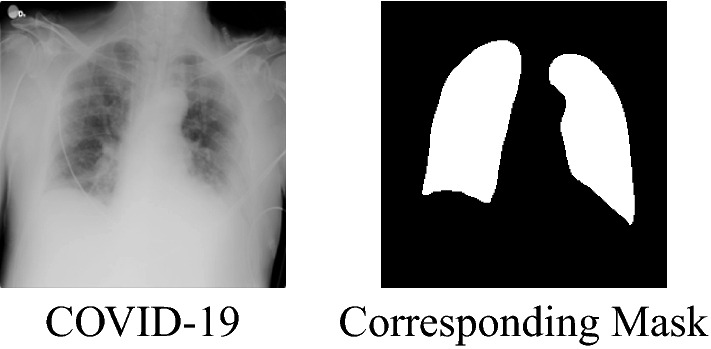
Example chest X-ray. This is a sample X-ray from training data. The plot on the right is a 2D array with values of 1 representing lungs and 0 representing not lungs. This allows the mask to be multiplied with the original image element wise to crop out the lungs without the heart in the middle or the remaining X-ray
